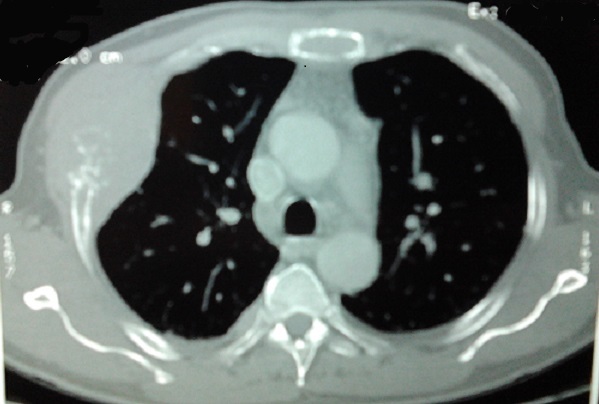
Chest computed tomography revealed right mass with lysed costal lesion
